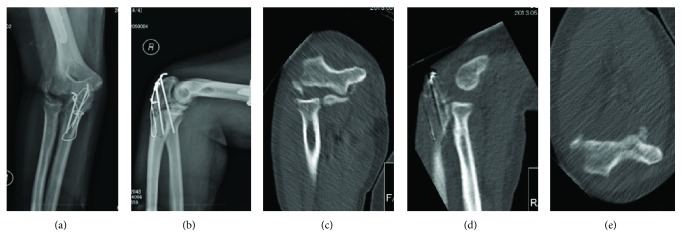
Radiographs and computed tomography (CT) image 3 months after surgery. (a) AP view and (b) lateral view of the elbow. The avulsed fracture of the lateral epicondyle is displaced, the radiohumeral joint is widened in the AP view, and the posterior subluxation of the radial head is widened in the lateral view. The joint congruency of the ulnohumeral joint is maintained. (c) CT image in the coronal plane, (d) sagittal view, and (e) axial view. The displaced lateral epicondylar fracture is malunited.
